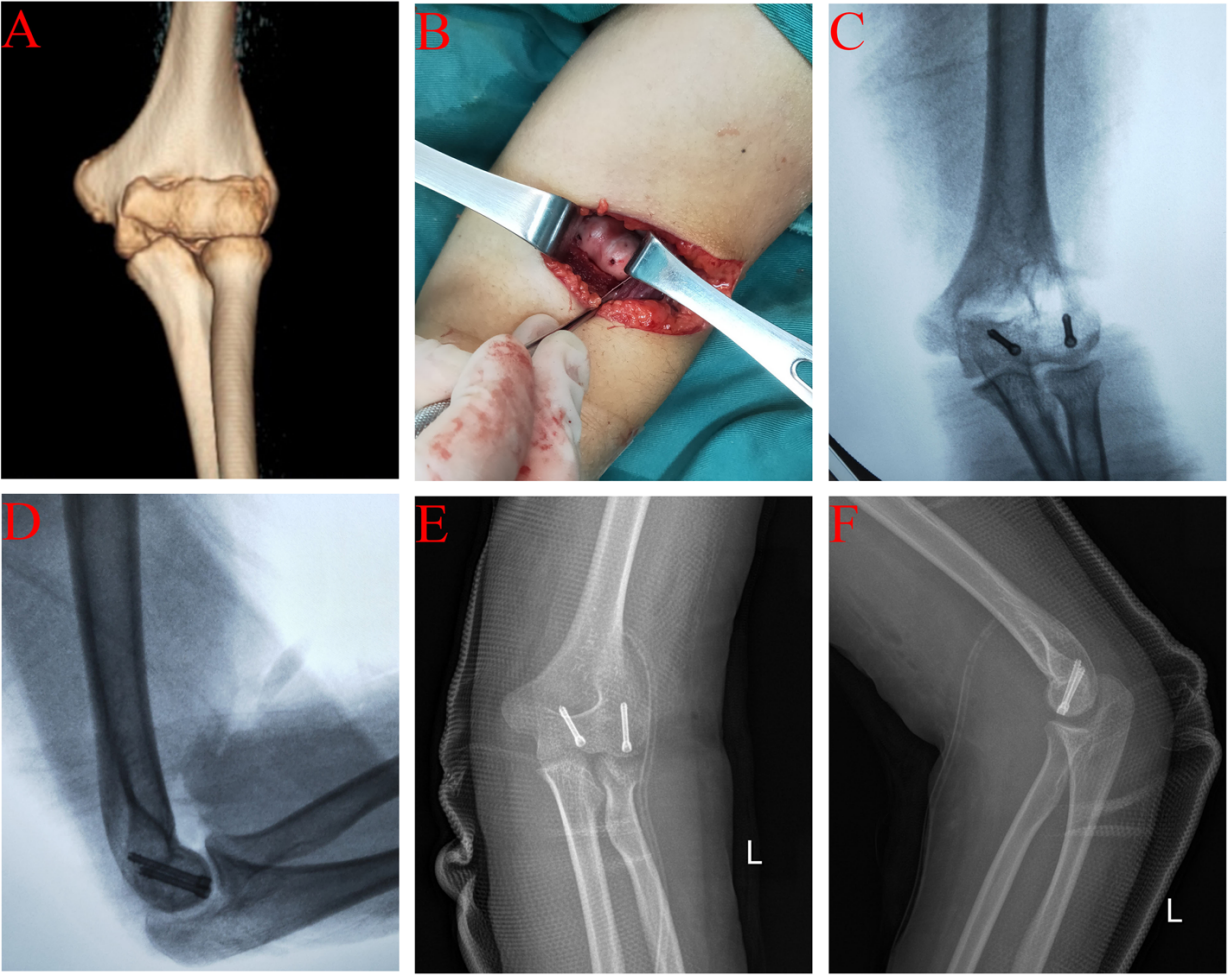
A 13-year-old girl presented with swelling, pain, and dysfunction of the left upper limb due to a fall. CT of the elbow joint showed a capitellar fracture (A). She underwent reduction of the capitellar fracture via the anterior approach under direct vision. Herbert screws were used to fixate the fracture fragments (B). C-arm fluoroscopy (C anteroposterior view of the elbow joint; D lateral view of the elbow joint) confirmed satisfactory reduction and fixation of the fractures. Follow-up radiography was performed on the first day after surgery. The anteroposterior view (E) and lateral view (F) showed that the reduction and fixation were good
